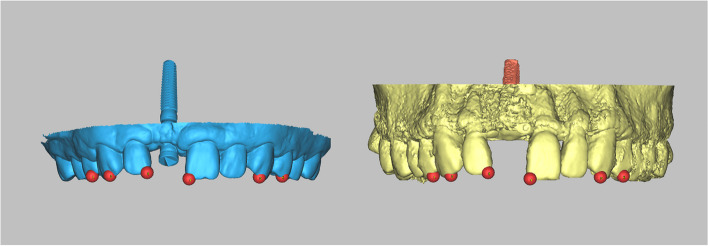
The multi-point registration approach was employed to register the “STL-IOS with implant” with the “STL-CBCT”
